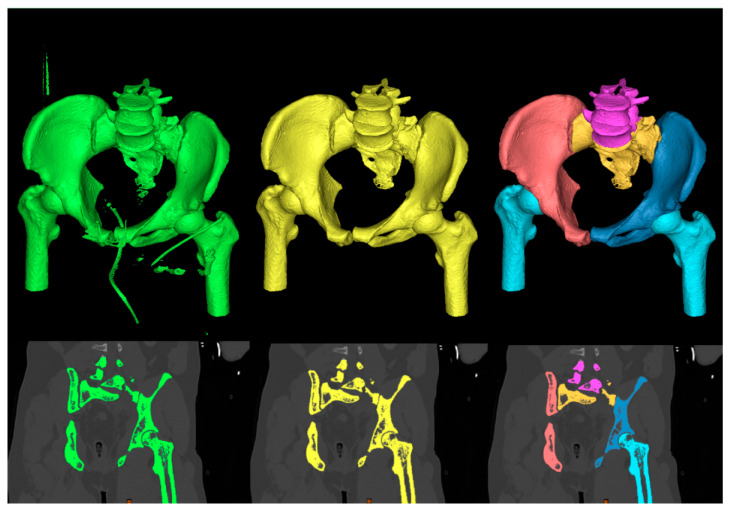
Process of segmenting and separating pelvis from CT slices into individual anatomical structures. Left to right: thresholding output (green color), region growing output (yellow color) and mask splitting output (multiple colors). The top row is a representation of masks in 3D space, and the bottom row is in 2D space.
